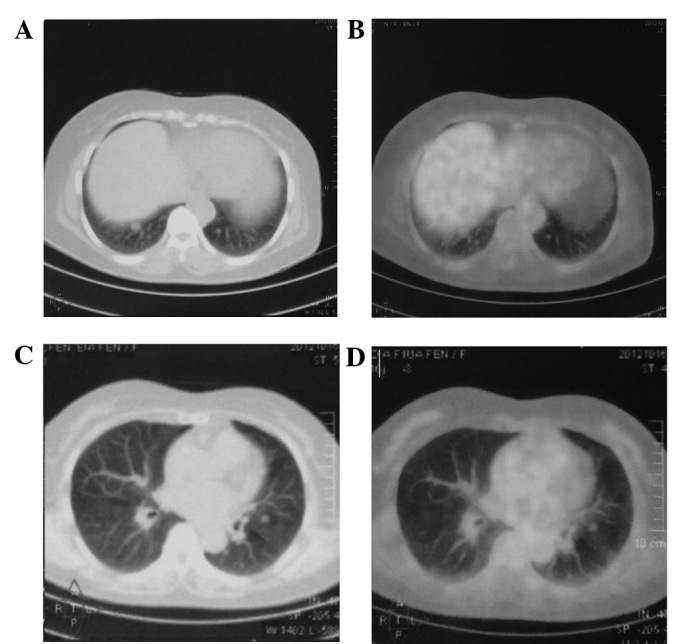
(A and C) Computed tomography (CT) and (B and D) ^18^F-fluoro-2-deoxy-D-glucose (FDG)-positron emission tomography/CT scan images exhibit a mild uptake of ^18^F-FDG in the pulmonary nodule indicative of malignancy.
